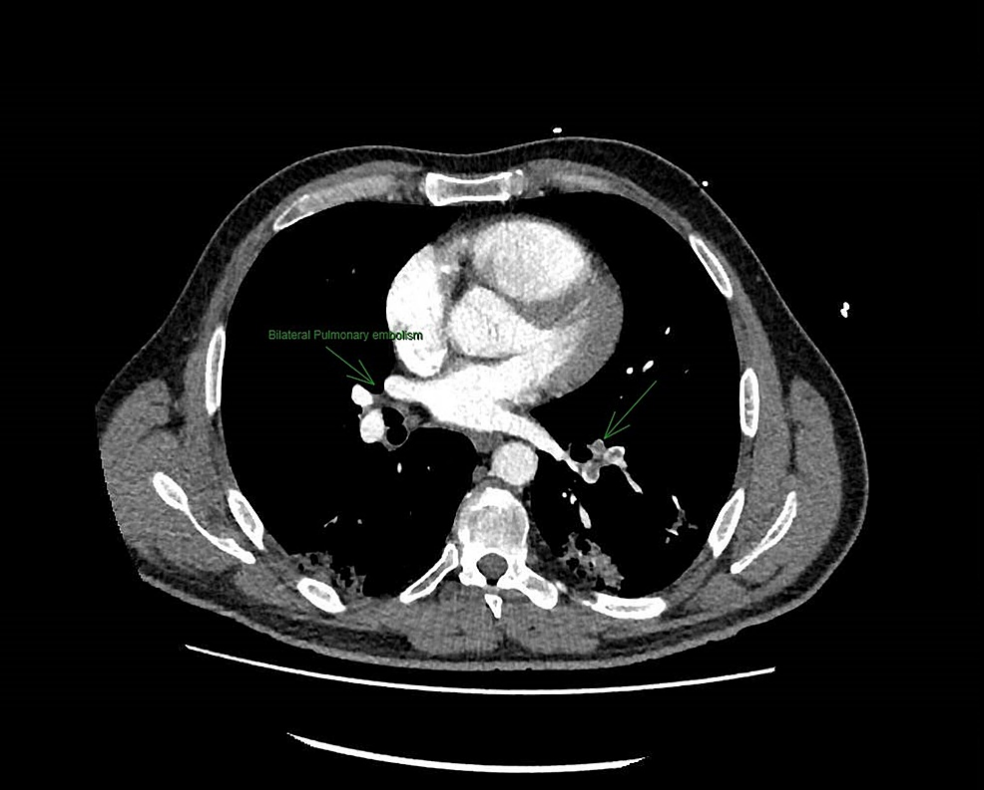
CTPA showing large bilateral pulmonary embolism CTPA: computed tomography pulmonary angiography
